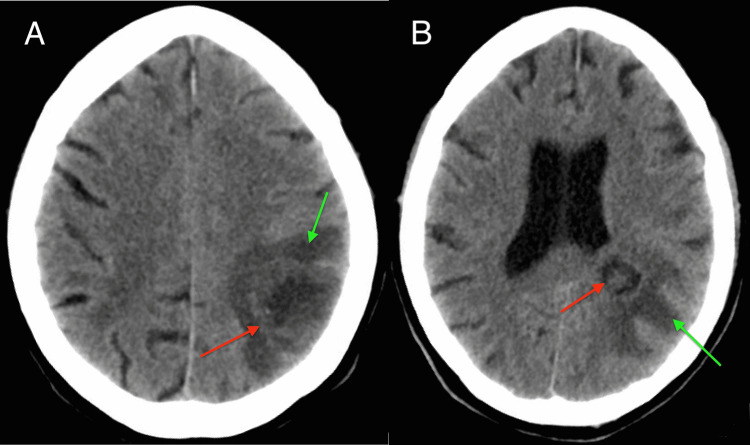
Computed tomography head without intravenous or oral contrast, axial view. Red arrows show a centrally necrotic lesion with internal heterogeneity in the left parietal lobe (A) extending towards the left lateral ventricle (B) with surrounding vasogenic edema (green arrows) and localized mass effect. It measures 3.5 x 2.5 x 2.5 cm. This is suggestive of a pyogenic or atypical abscess or a necrotic/cystic tumor.
